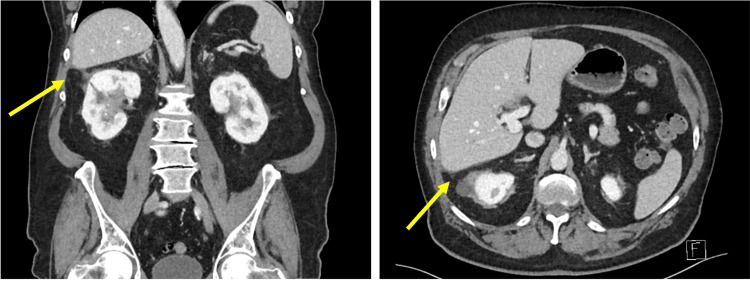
Computed tomography (CT) scan performed after removal of the surgical drain within the perihepatic collection. Portal venous phase CT images demonstrated complete resolution of the previous perihepatic collection (yellow arrows) in coronal (A) and axial (B) views.
